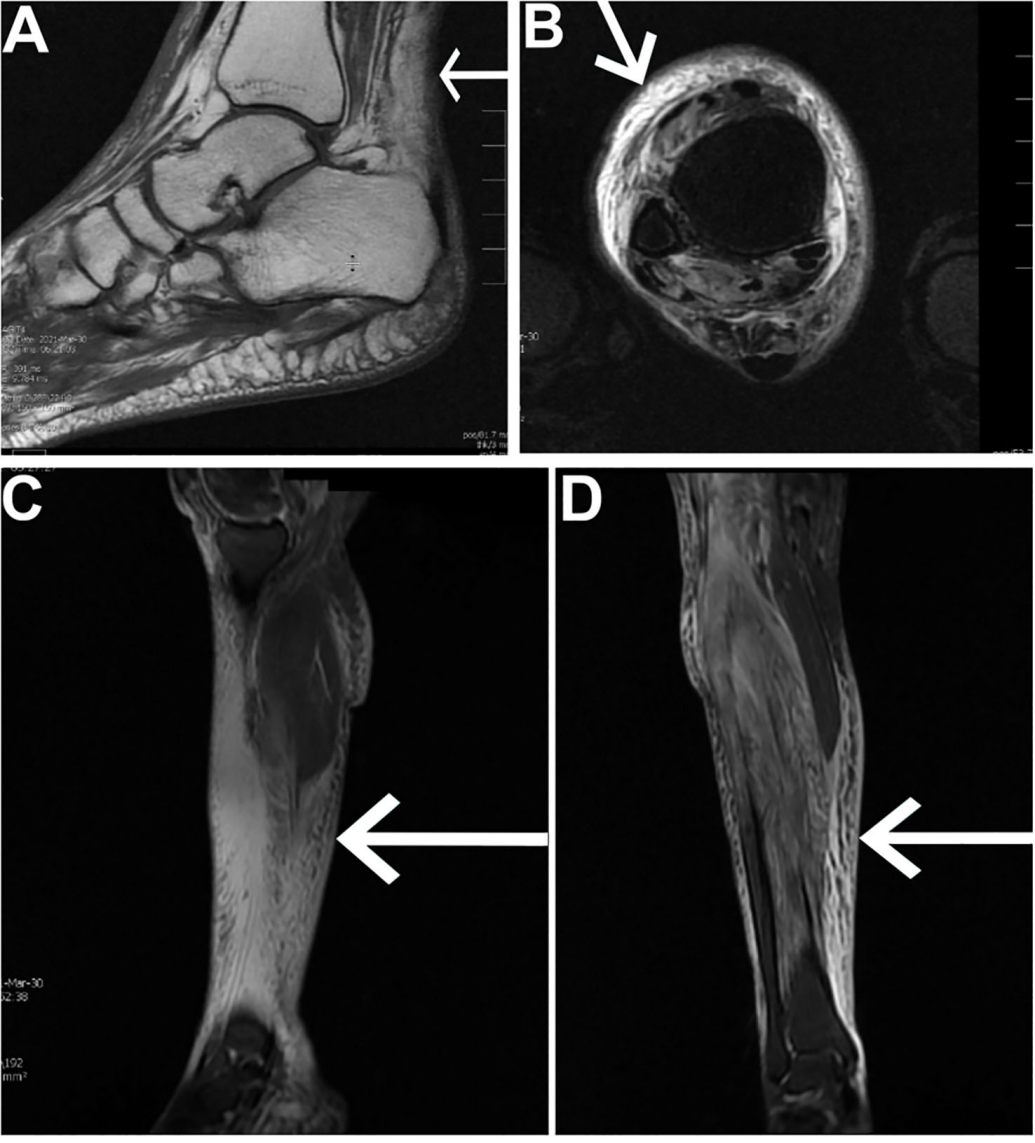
MRI of the right lower extremity. (A) T1-weighted sagittal view of the right ankle and foot demonstrating diffuse muscle and subcutaneous edema. (B) T2-weighted/short-tau inversion recovery (STIR) axial view of the right lower extremity at the level of the ankle demonstrating diffuse inflammation. (C) T2-weighted/STIR sagittal view of the right lower extremity demonstrating diffuse inflammation of the calf, most severe along the anterior–medial aspect. (D) T2-weighted/STIR coronal view of the right lower extremity demonstrating diffuse inflammation.
